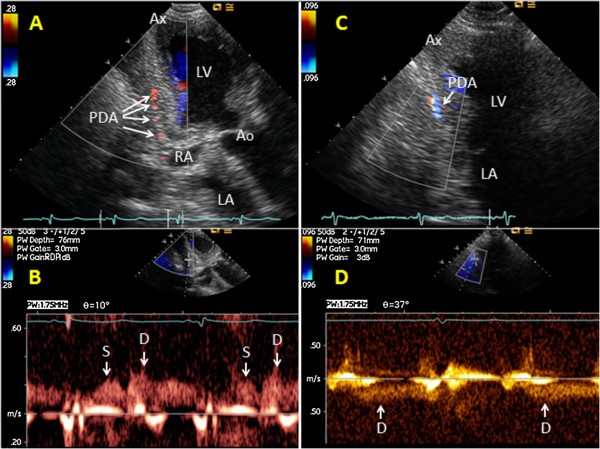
Examples of anterograde and retrograde flow in the posterior descending coronary artery. In modified apical long-axis views focusing on the posterior interventricular sulcus, the posterior descending coronary artery (PDA) is imaged by colour Doppler mapping with matching spectral Doppler tracings of blood flow: (A,B) The PDA is seen with anterograde flow. (C,D) The PDA is seen with retrograde flow. Ao = aortic valve and ascending aorta, Ax = apex, D = spectral Doppler tracings of diastolic coronary blood flow, LA = left atrium, LV = left ventricle, RA = right atrium, S = spectral Doppler tracings of systolic coronary blood flow.
